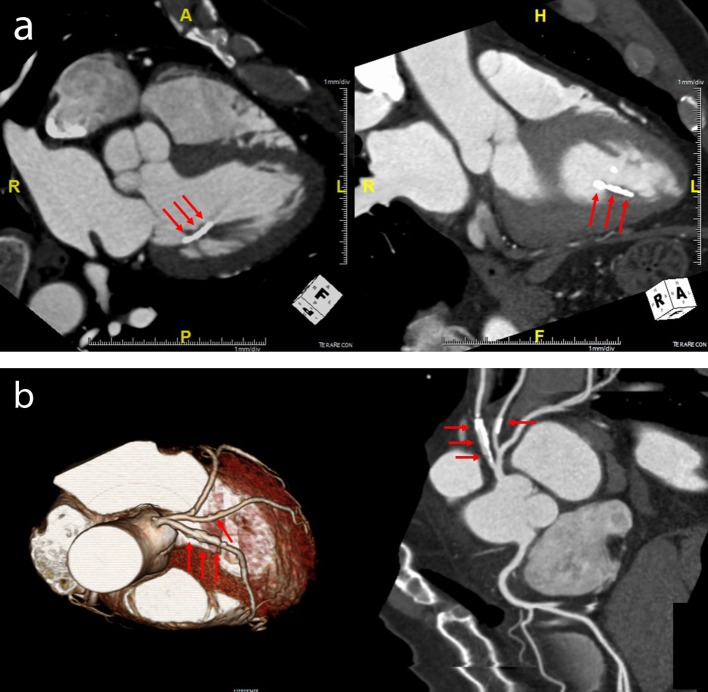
(a) Multidetector computed tomography demonstrated a spindle-like extensive calcification of anterolateral papillary muscle (arrow); (b) diffuse mild stenosis with calcified plaques in proximal portion of left anterior descending coronary artery disease and mild segmental stenosis with calcified plaques in proximal portion of obtuse marginal artery (arrow).
